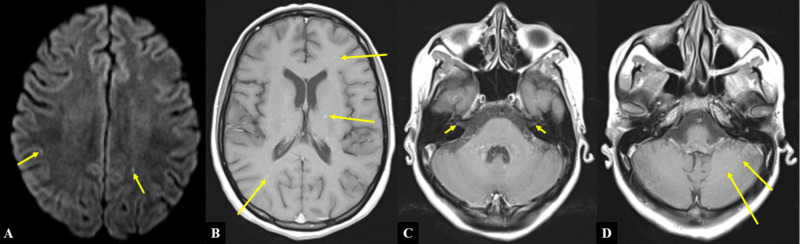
MRI of the brain A) Diffusion-weighted image of bilateral cerebral hemispheres. B-D) T1-weighted images of selected coronal sections in superior-to-inferior order. Arrows indicate locations of select hyperintense lesions. Note that not all lesions are identified by the arrows MRI: magnetic resonance imaging
